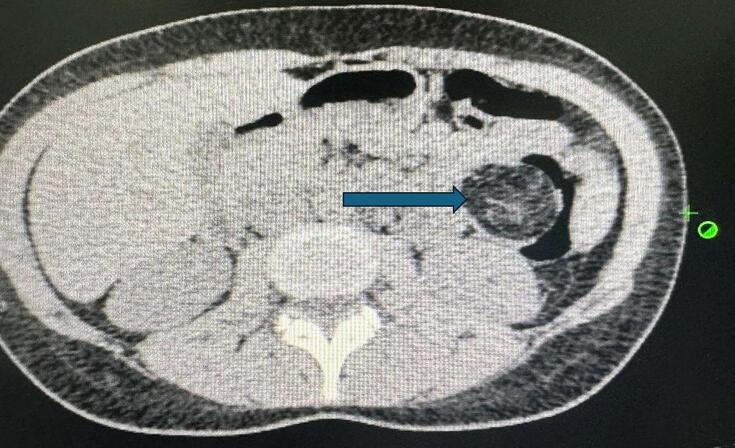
CT scan of the abdomen showing: a round well-defined 3.8 × 3.4 cm mass in the proximal aspect of the descending colon, with most likely lipid in nature.
